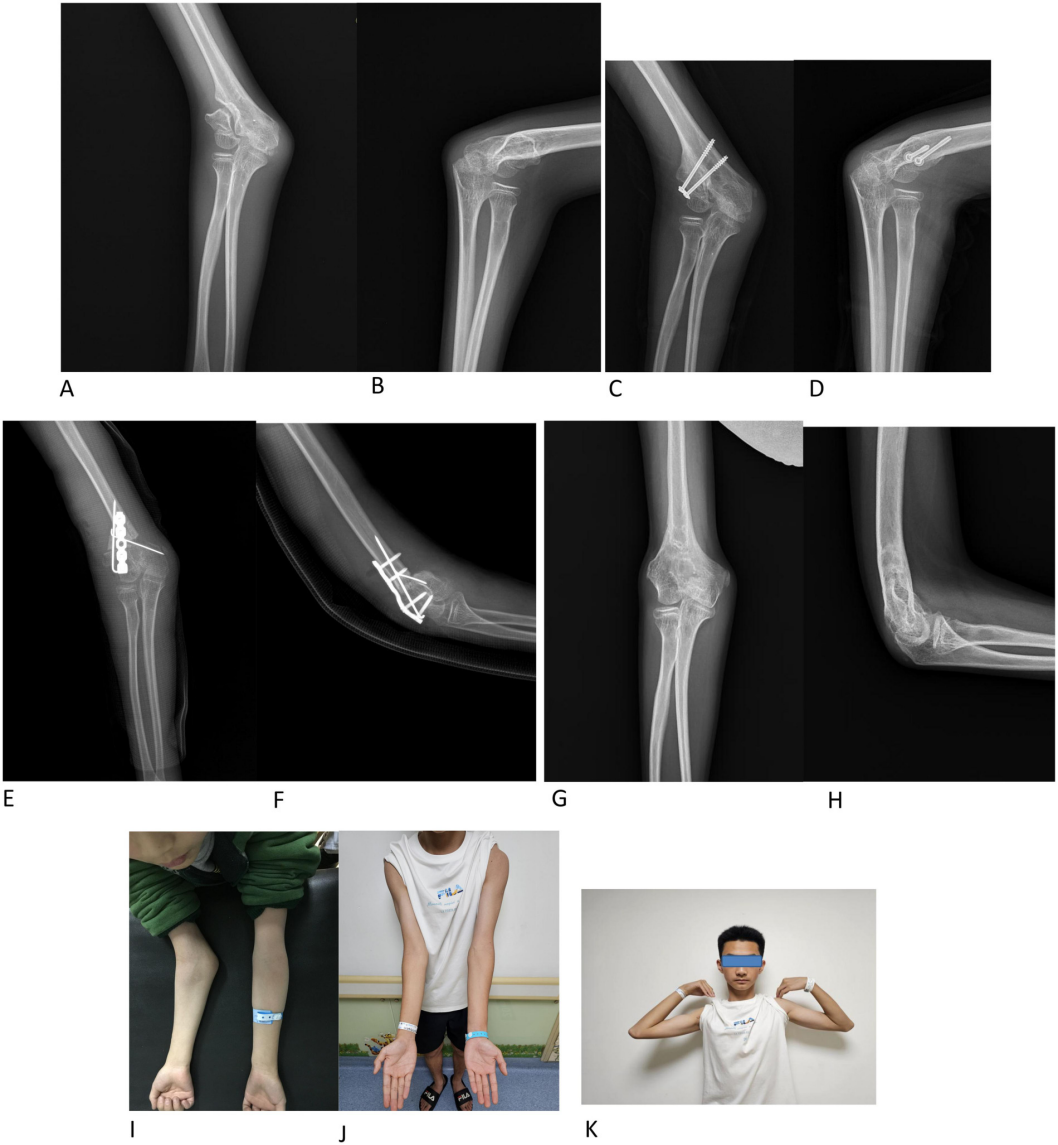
A 11-year-old with an old humeral condylar fracture of his right elbow. A–B: radiographs showing non-union of the old humeral condylar fracture and cubitus valgus. C–D: the first stage of surgery: internal reduction and use of 2 4.0 mm hollow compression screws to fix the block. E–F: the second stage of surgery: osteotomy of supracondylar humerus to correct cubitus valgus and internal fixation with a 5-hole reconstruction locking plate and 2 K-wires. G-H: radiographs one year after the second operation and after plate and K-wire removal. I: severe cubitus valgus before operation. J-K: normal appearance and function of the left elbow.
